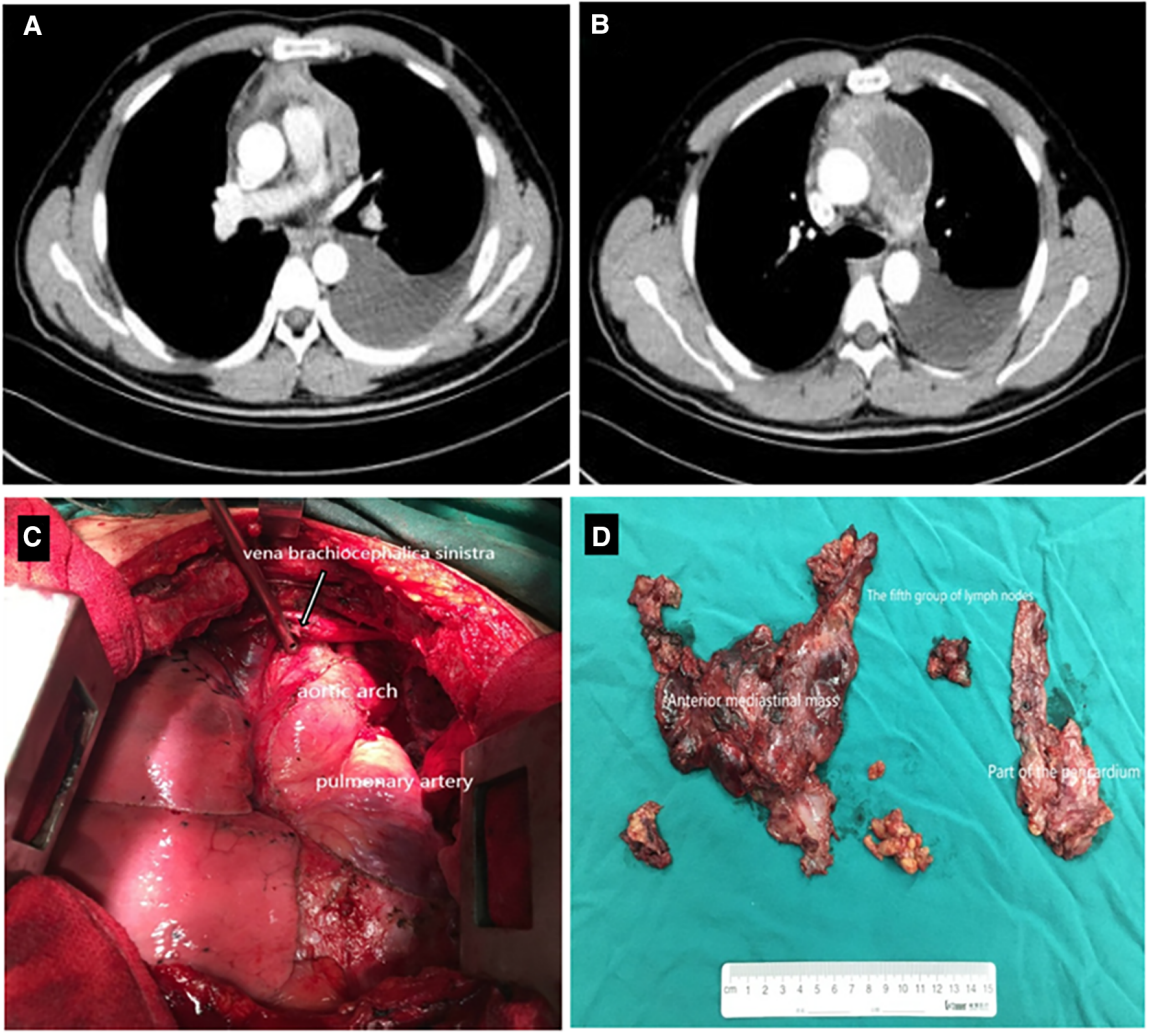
Contrast-enhanced CT scan demonstrated a 7.4 × 4.9 cm^2^ heterogeneous mass in the anterior mediastinum, where a large area cystic liquid existed, and multiple enlarged lymph nodes in the mediastinum (A,B); The whole mass, invaded tissues and adjacent enlarged lymph nodes were removed completely (C,D). CT, computed tomography.
